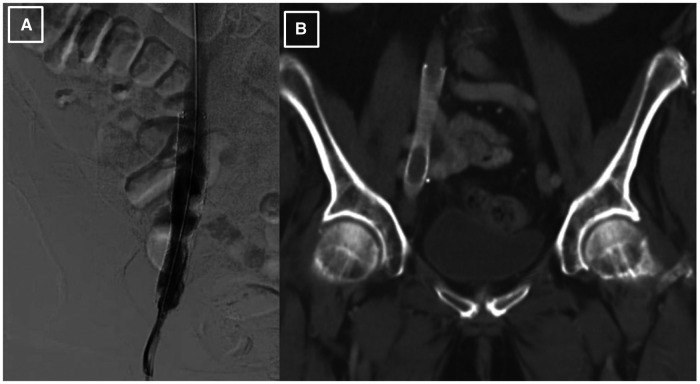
Post-procedural imaging of the stented right external iliac vein. (A). Iliac venography status post IVUS-guided visualization and right external iliac vein balloon-angioplasty with successful placement of VICI 14 × 90 mm stent and resultant patency. (B). CT abdomen pelvis demonstrating presence of stent within the R external iliac vein prior to abdominal debulking procedure.
